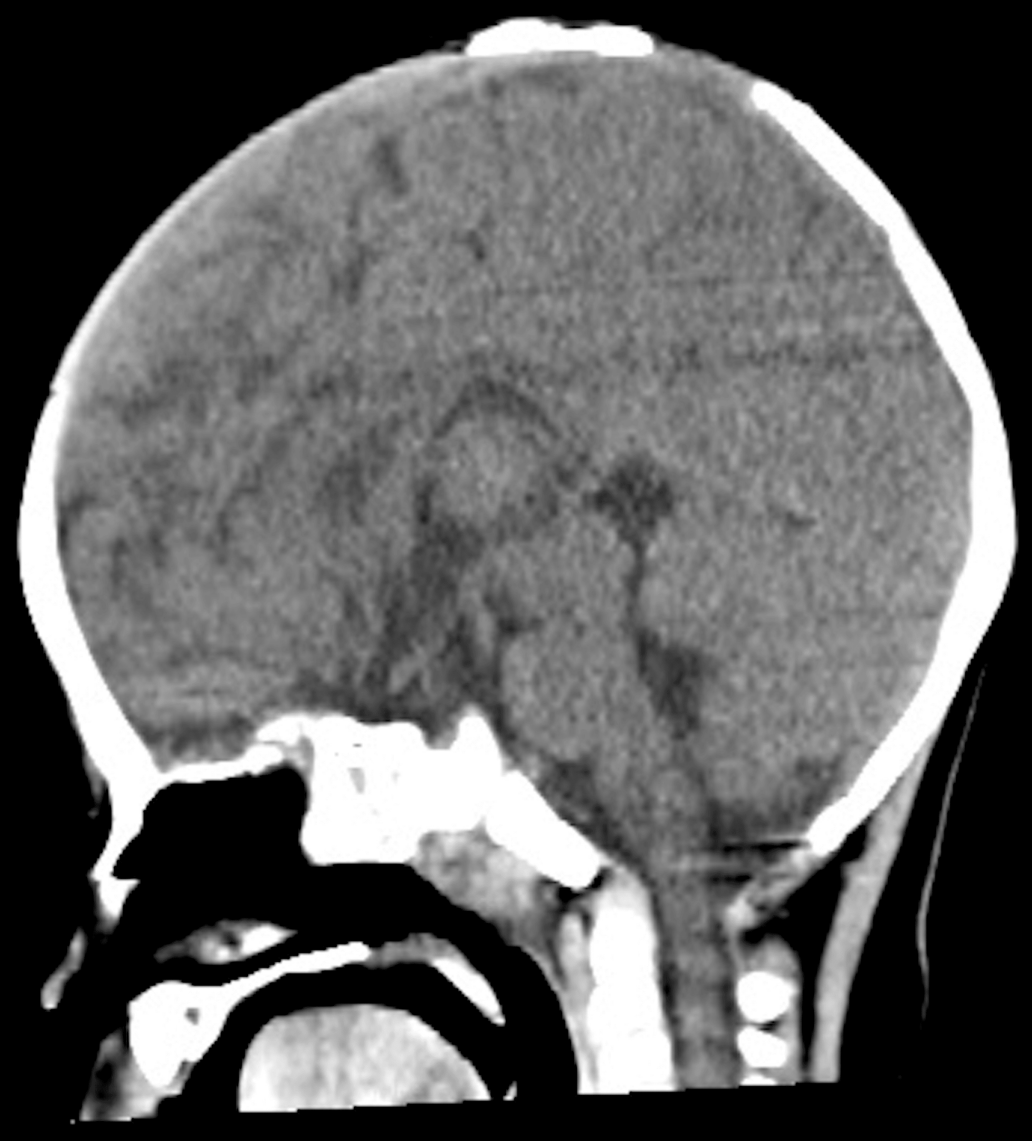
Non-contrast head CT (sagittal view) at 10 months of age. The anterior fontanelle is markedly enlarged, with delayed suture closure.
